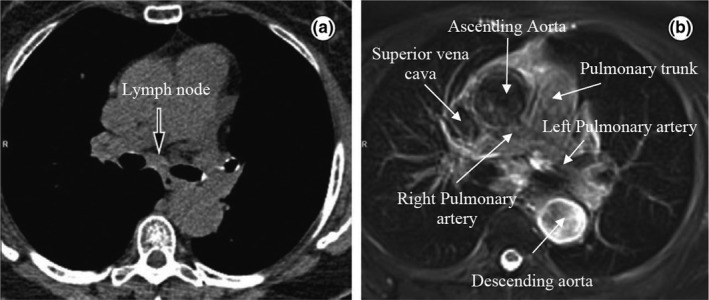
Axial slices of the mediastinum showing (a) a CT image and the corresponding (b) T_2_ weighted MRI image with improved soft‐tissue contrast. Some structures (the great vessels) are highlighted in the MRI image. Reprinted from Hochhegger et al.^26^
